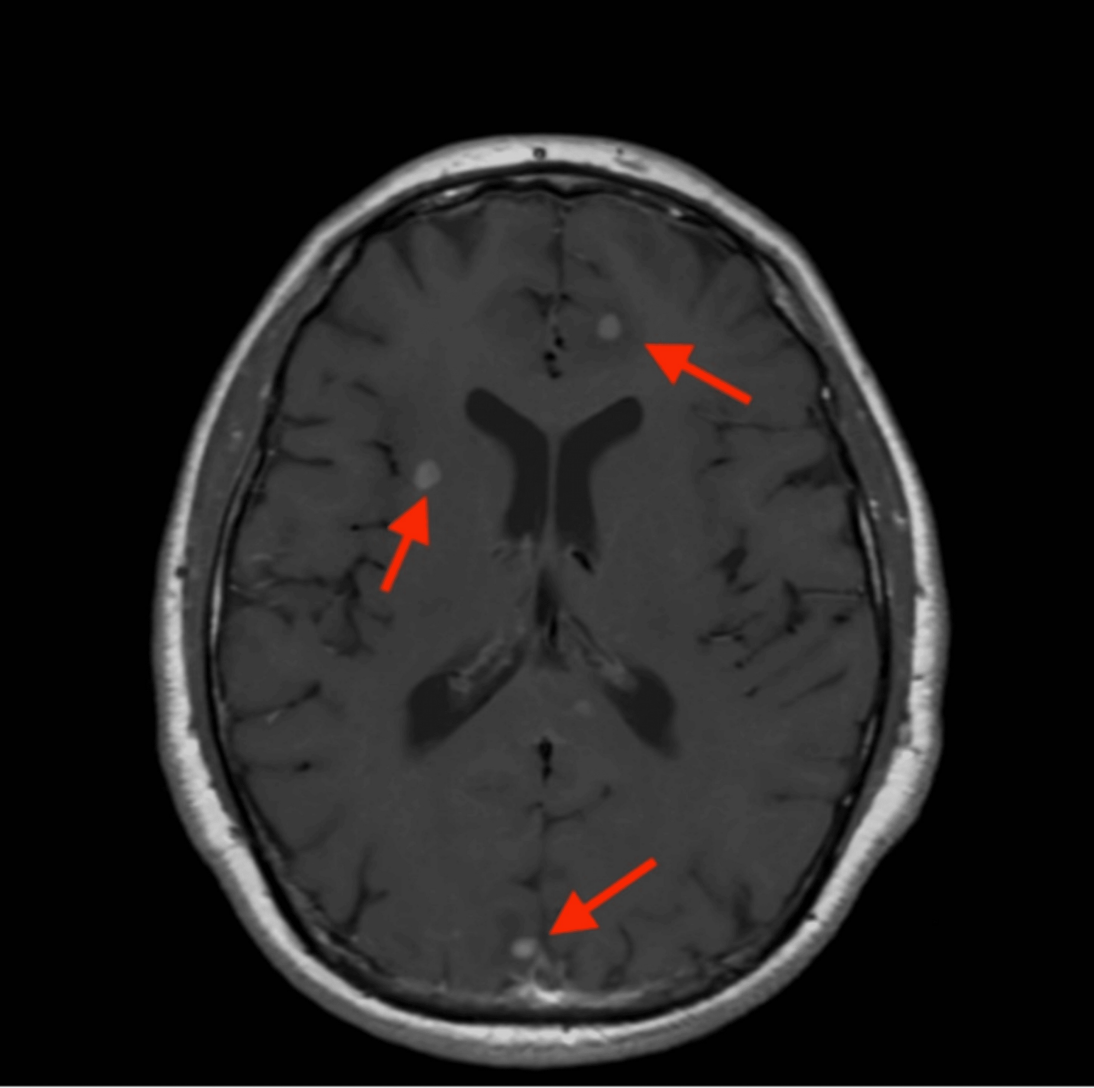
Brain MRI: axial FLAIR The image demonstrates multifocal enhancing lesions with surrounding edema, consistent with an inflammatory or granulomatous process. MRI: magnetic resonance imaging; FLAIR: fluid-attenuated inversion recovery
